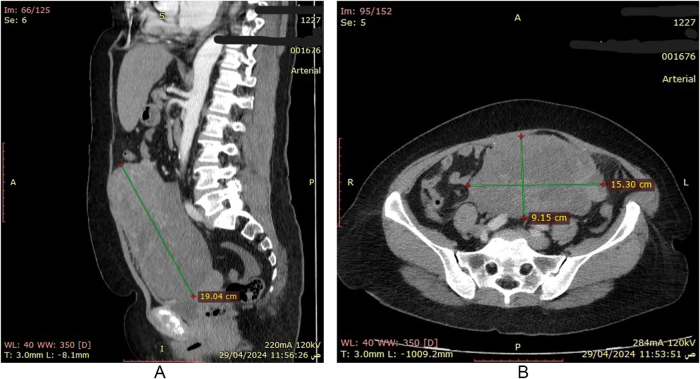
(A), (B) Axial and coronal views of contrast-enhanced CT scan before surgery showing a large, heterogeneous pelvic mass with central necrosis (arrows), initially suspected to be of uterine origin.
